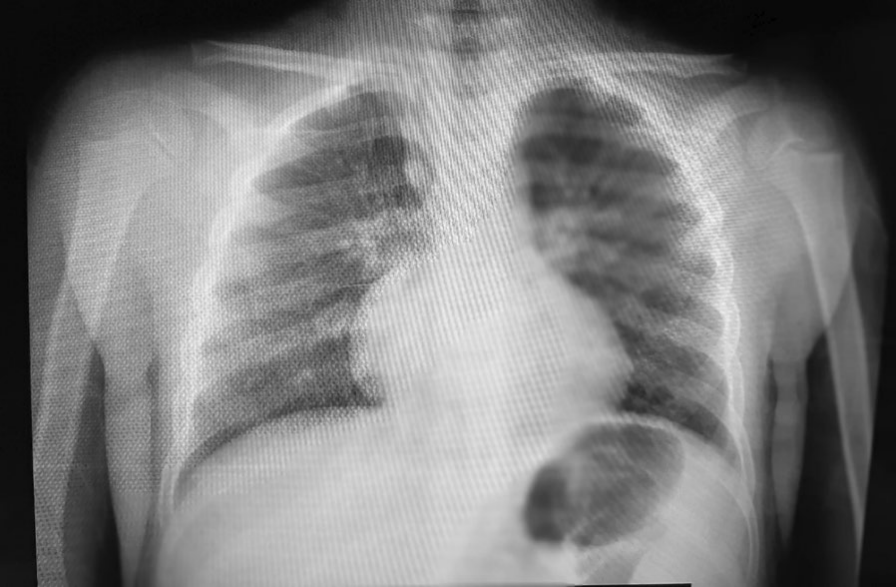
Frontal chest radiography shows bilateral minimal peripheral patchy opacities. The lung ultrasonography showed basal B lines, minimal bilateral basal pleural thickening, and minimal pleural effusion in both phrenicocostal sinuses
